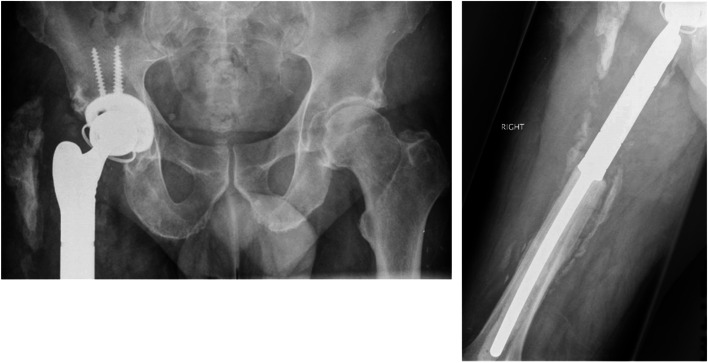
Radiographs of a patient with a Proximal Femoral Replacement taken prior to DAIR surgery
